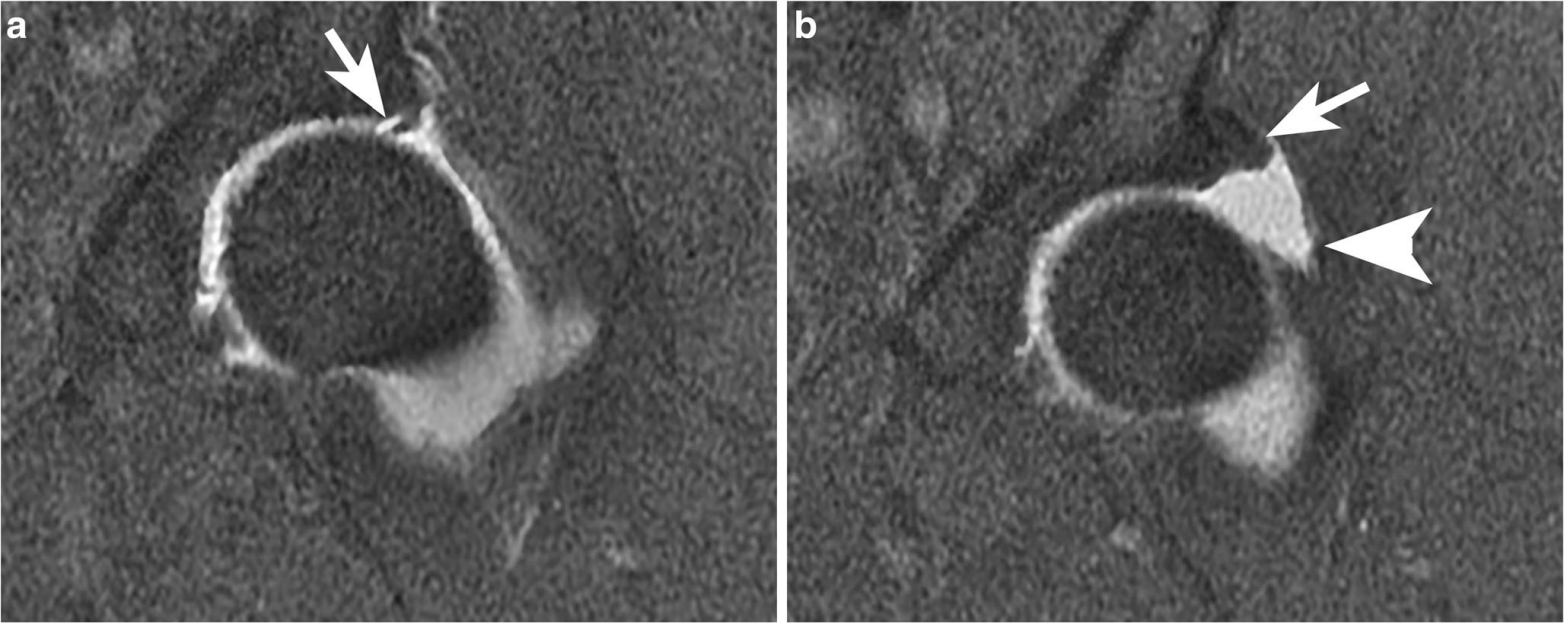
A 35-year-old woman with labral displacement after surgery. a Preoperative coronal T1-weighted fat-saturated T1FS MR arthrogram at 1.5 T shows a tear of the anterosuperior labrum (*arrow*). b Postoperative coronal T1FS MR arthrogram at 1.5 T shows that the labrum has displaced superiorly (*arrow*) and is adherent to the lateral margin of the ilium immediately above the articular surface. Capsule defect (*arrowhead*) is also evident
